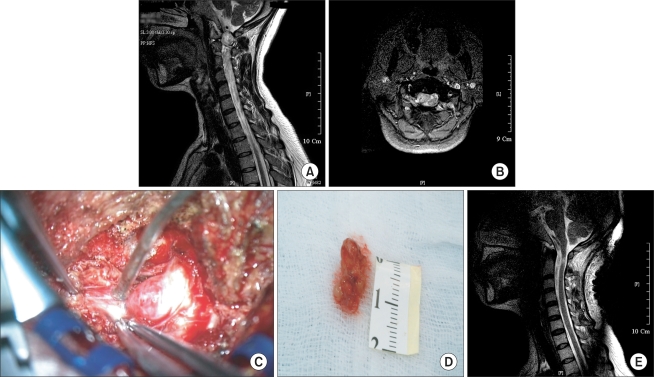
Thirty-five-year-old male with intradural extramedullary schwannoma with both upper & lower extremity weakness and tingling sensation below C3 level. (A) T2-weighted sagittal MR image shows a huge homogenous high signal intensity mass. The tumor is located at the C1-2 level. (B) T2-weighted coronal MR image shows a space occupying mass compressing the spinal cord severely. (C) Intra-operative findings of intradural extramedullary mass excision. (D) Extracted tumor showing a well encapsulated mass measuring 1.6 × 1 × 0.8 cm. (E) Postoperative MR image shows mass lesion removed at the C1-2 level, but the spinal cord signal changes remain.
